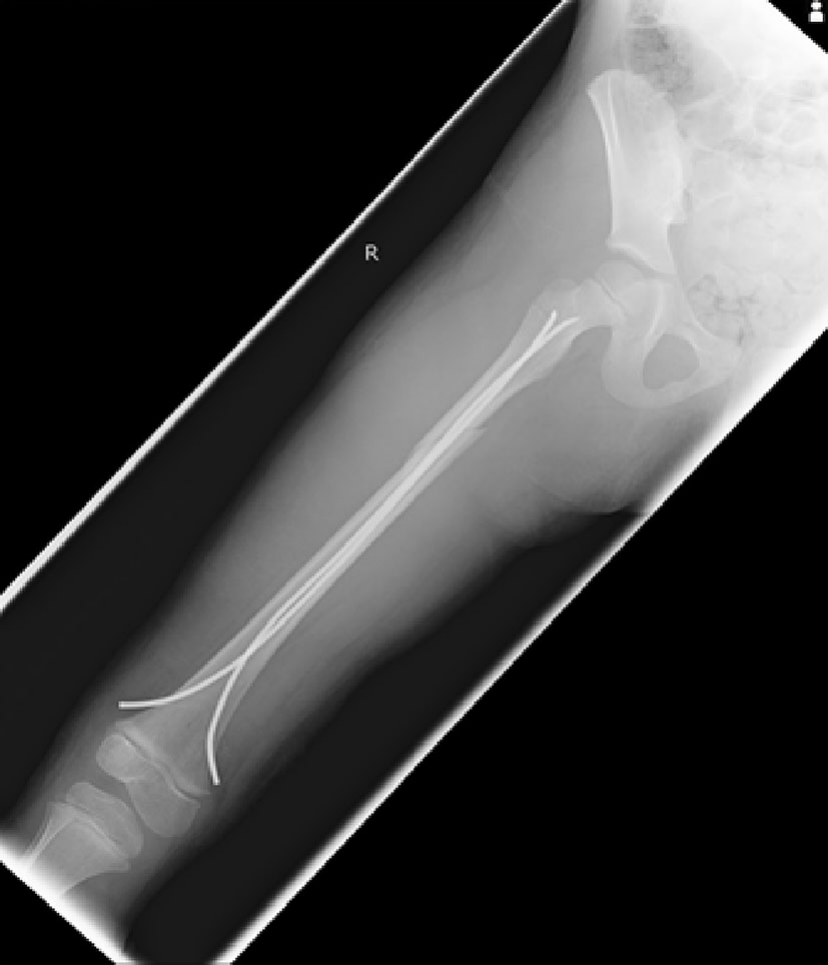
AP radiograph showing satisfactory restoration of length, rotation and alignment with the use of titanium elastic nails, of the fracture in Fig. 10
